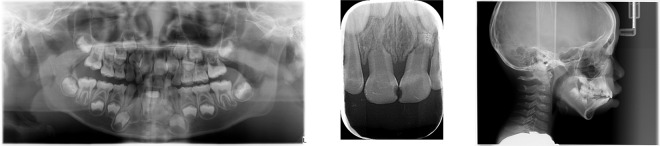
The figure shows three types of dental images we collected from hospitals, all of which are important tools for dentists to diagnose dental diseases. From left to right: dental panoramic radiographs, intraoral scan, CBCT.
